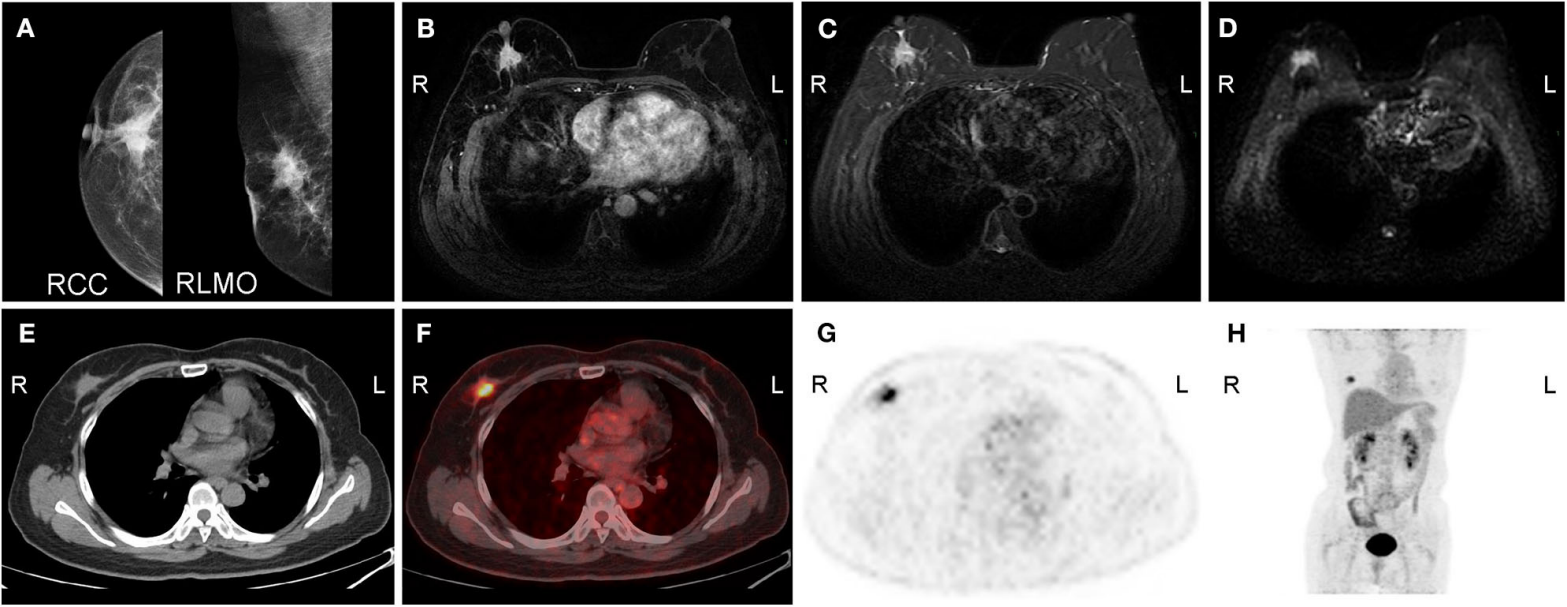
A 50-year-old woman with carcinoma of the right breast. (A) Molybdenum target mammography shows a mass in the upper quadrant of the right breast with an irregular shape, fuzzy boundary, speculation, linear calcification, and a retracted nipple. (B) Enhanced MRI shows that the right breast mass was significantly enhanced during the arterial phase, with a high signal on (C) T2WI/FS and (D) DWI. Axial (E) CT, (F) fused image, (G) FDG-PET, and (H) MIP (maximal intensity projection) image show irregular nodules with intense uptake on the upper outer quadrant of the right breast. R, right; L, left; RCC, right craniocaudal; RMLO, right mediolateral oblique.
